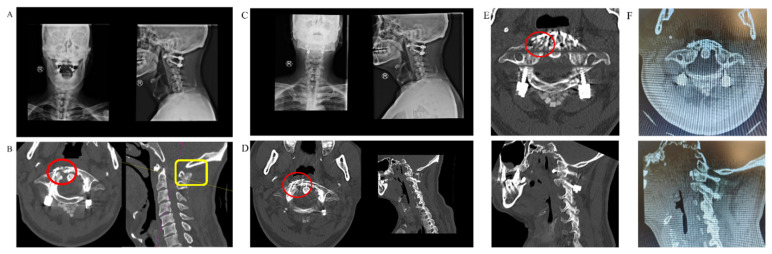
Follow-up imaging: (A) Anteroposterior and lateral cervical radiographs at 1 month postoperation. (B) Atlas CT at one month postoperation. (C) Cervical spine anteroposterior and lateral radiographs at two months postoperation. (D) Atlas CT at two months postoperation. (E) Atlas CT at three months postoperation. (F) Atlas CT at nine months postoperation. Red circle: fracture of the anterior atlas arch; yellow square: posterior atlantal bone graft region.
